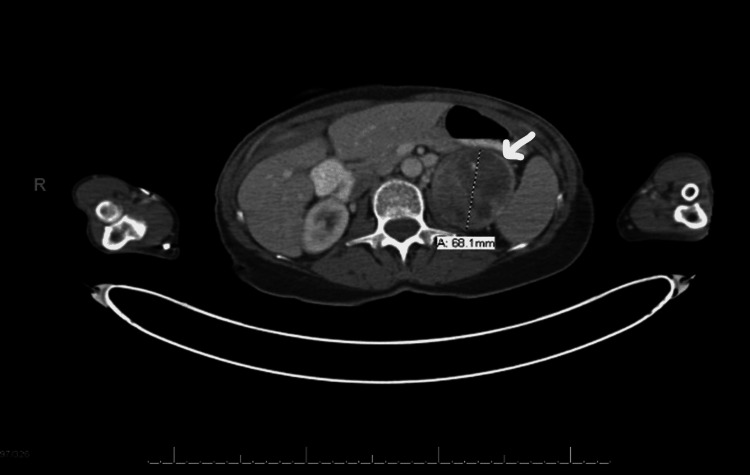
PET/CT image (preoperative) PET/CT: positron emission tomography/computed tomography
